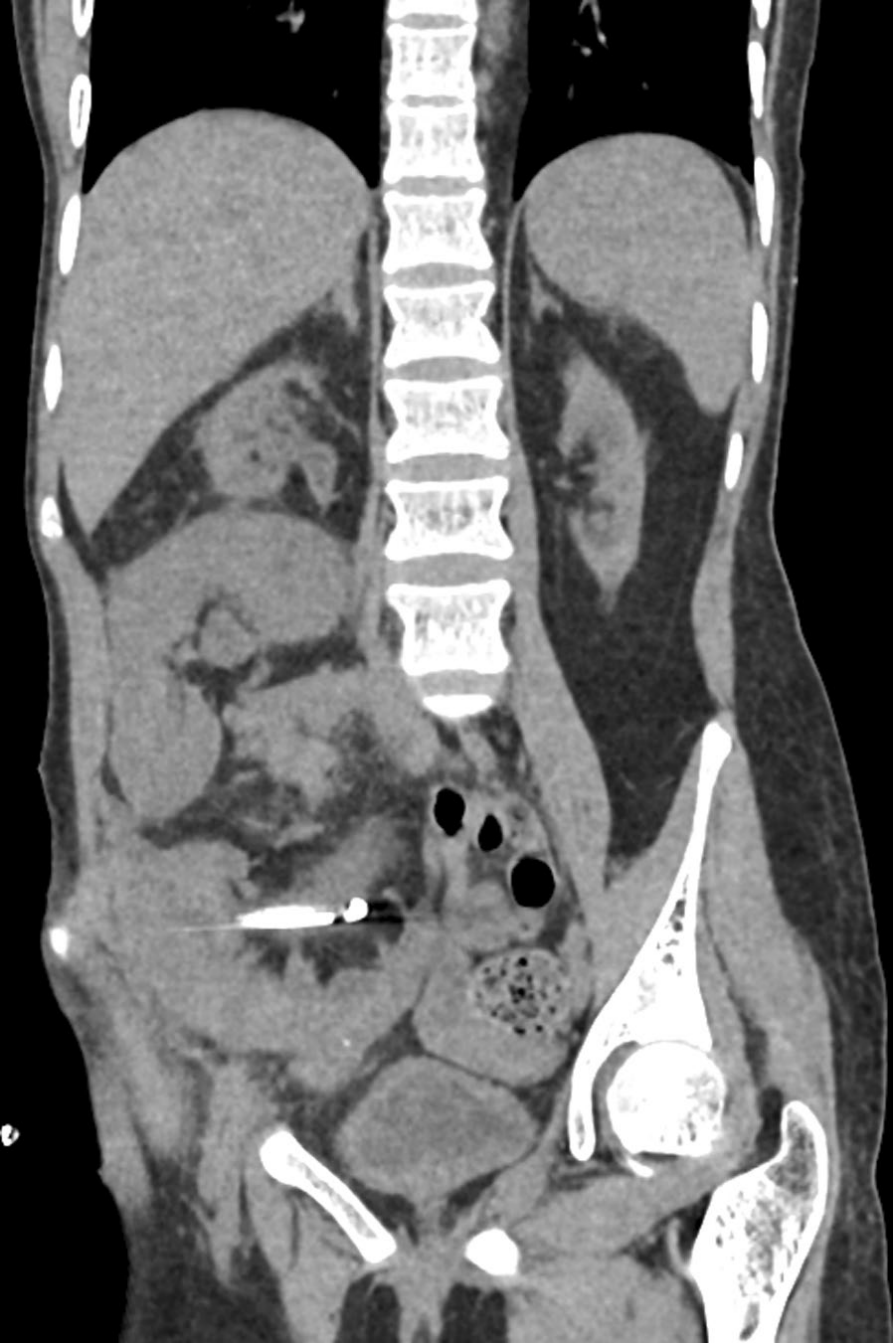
Postoperative CT scan without neither residual fragments nor subcapsular hematoma
